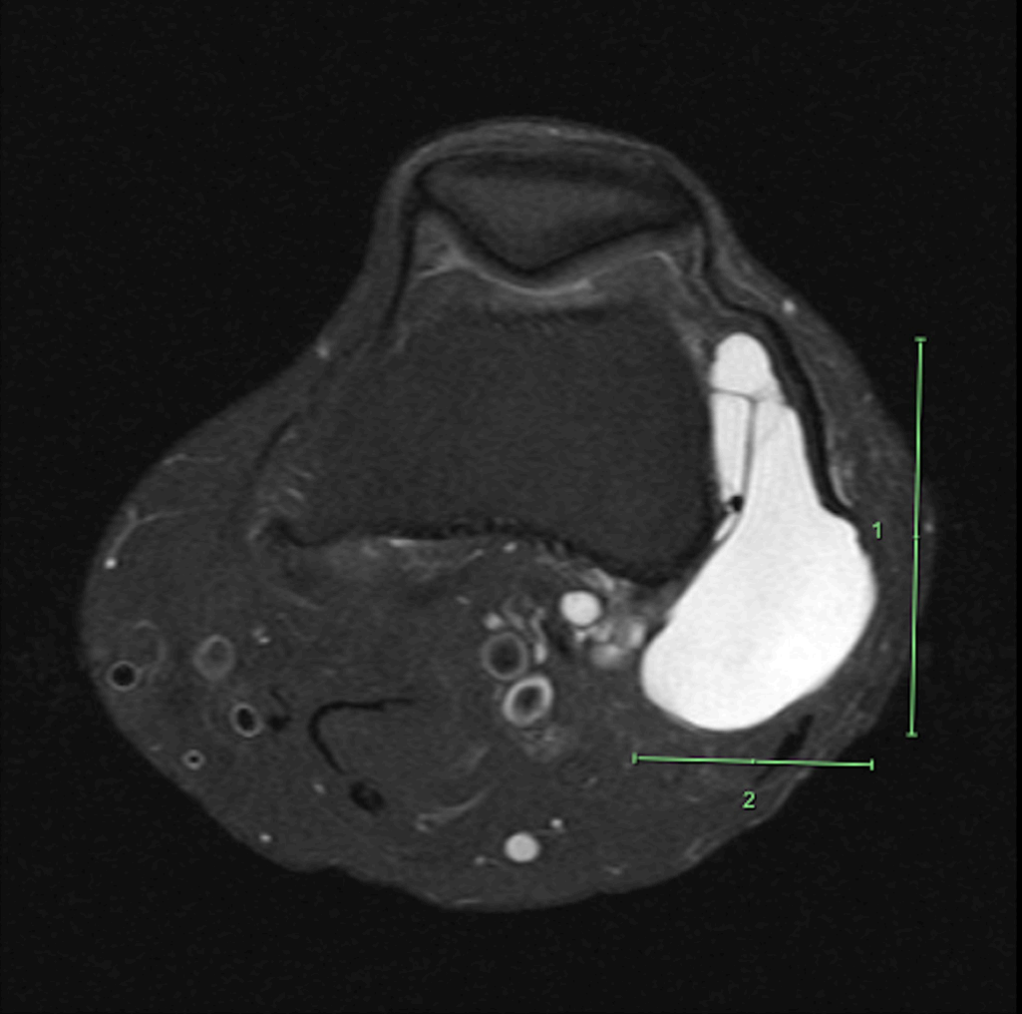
T2 axial view MRI without contrast of the left knee depicting multiloculated cystic lesion deep to the IT band.
